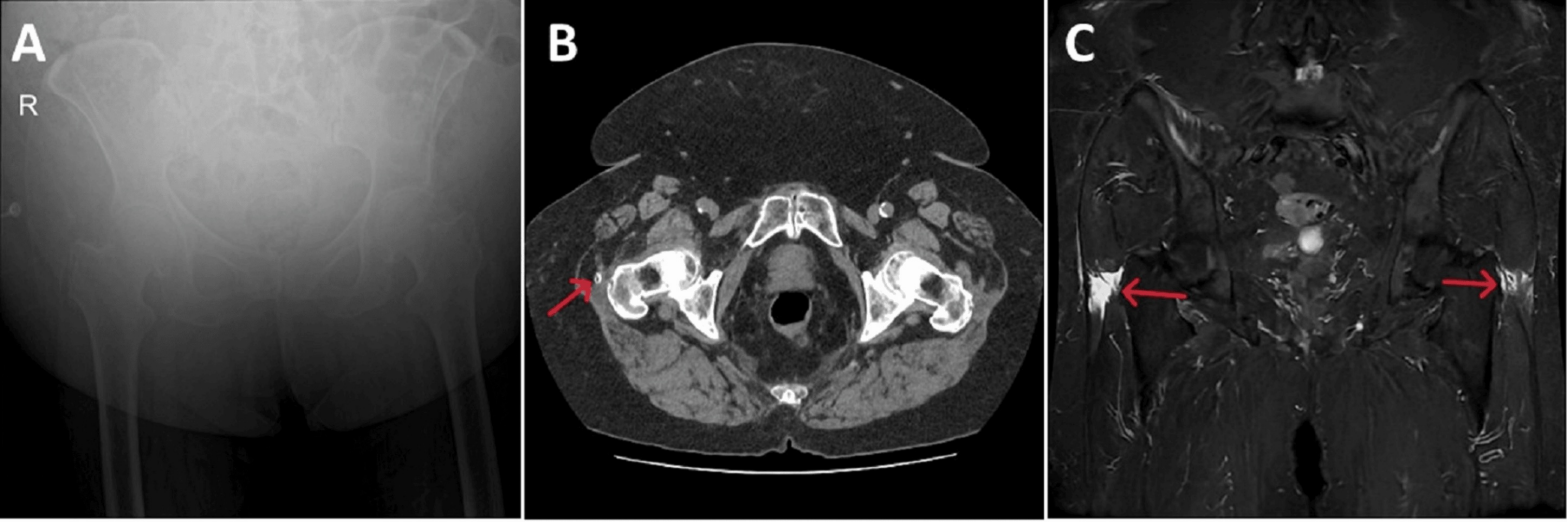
Radiological images of the pelvic, hip joint, and femur bones. A: Suboptimal X-ray film showing no fractures. B: Computed tomography scan of the pelvis at the level of the greater trochanter (transverse view) demonstrating enthesopathy near the right greater trochanter and some calcifications associated with the gluteus medius tendon (red arrow). C: Magnetic resonance scan of the pelvis at the level of the greater trochanter (coronal view) demonstrating bilateral gluteus maximus tendon tear with evidence of edema indicating that these were acute events (red arrows).
